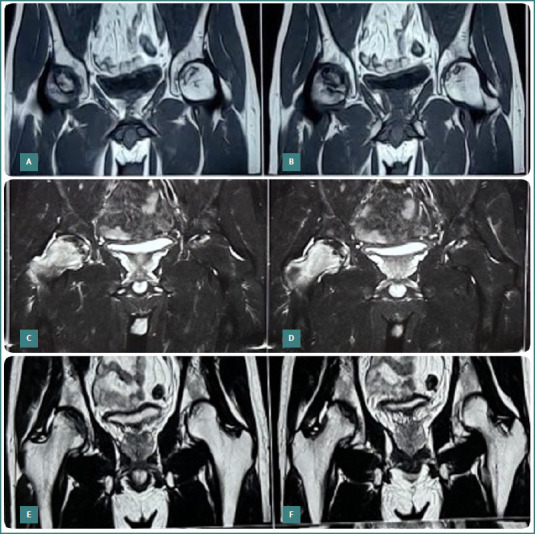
Serial MRI images of a patient treated with core decompression and autologous adult live-cultured osteoblast implantation. A–B, preoperative coronal MRI sections of the same patient with Ficat and Arlet stage IIb in the right hip and stage 2a in the left hip. C–F, MRI images at 24 months follow-up showing increased osteogenesis and signs of healing.
